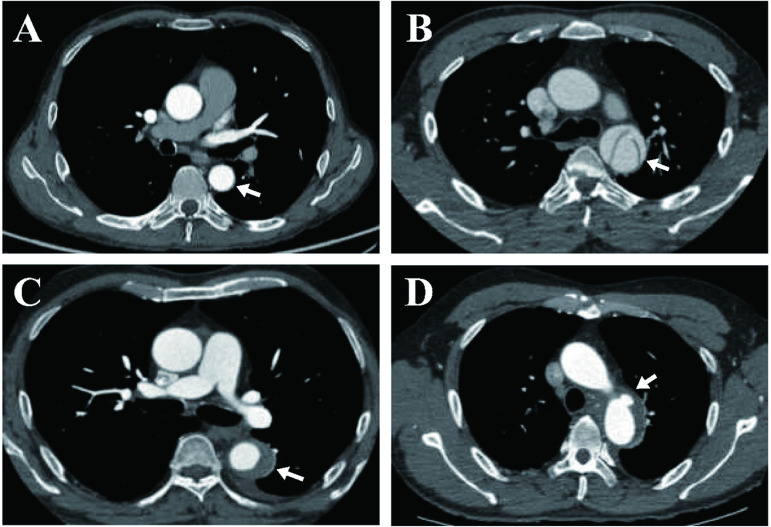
Representative CT angiography images of different types of acute aortic syndromes are shown. (A) Normal aorta; (B) Aortic dissection; (C) intramural hematoma; (D) Penetrating aortic ulcer. The arrows indicate the aorta.
